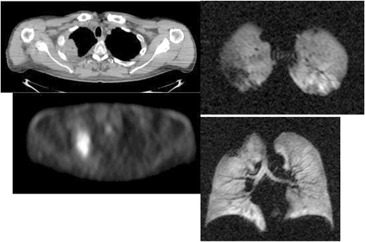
Following XRT, response is seen on CT, PET and Helium MRI.
